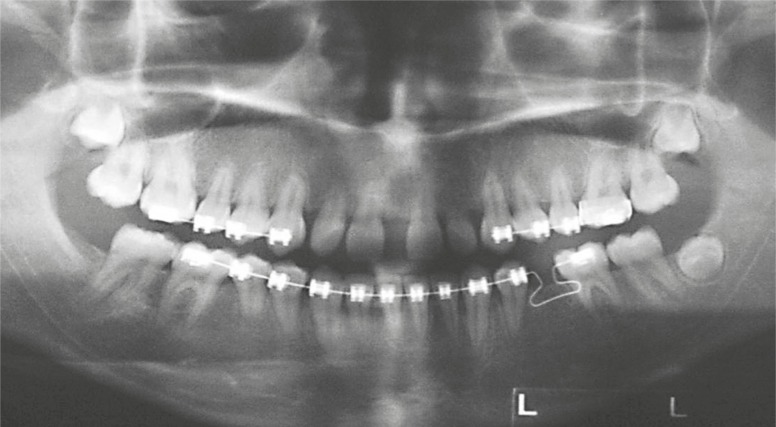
Control panoramic radiograph.
